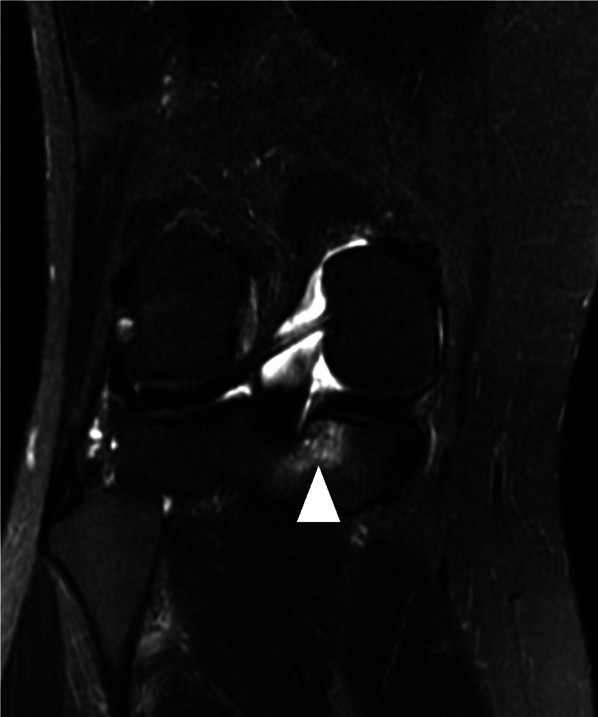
Representative magnetic resonance image showing spreading root sign. Representative SRS images in the coronal plane are shown. High signal intensity is observed in the subchondral bone lesion along with the attachment of the medial meniscus posterior root on T2-weighted fat saturation fast spin-echo images. The white arrow indicates SRS. *SRS* spreading root sign
